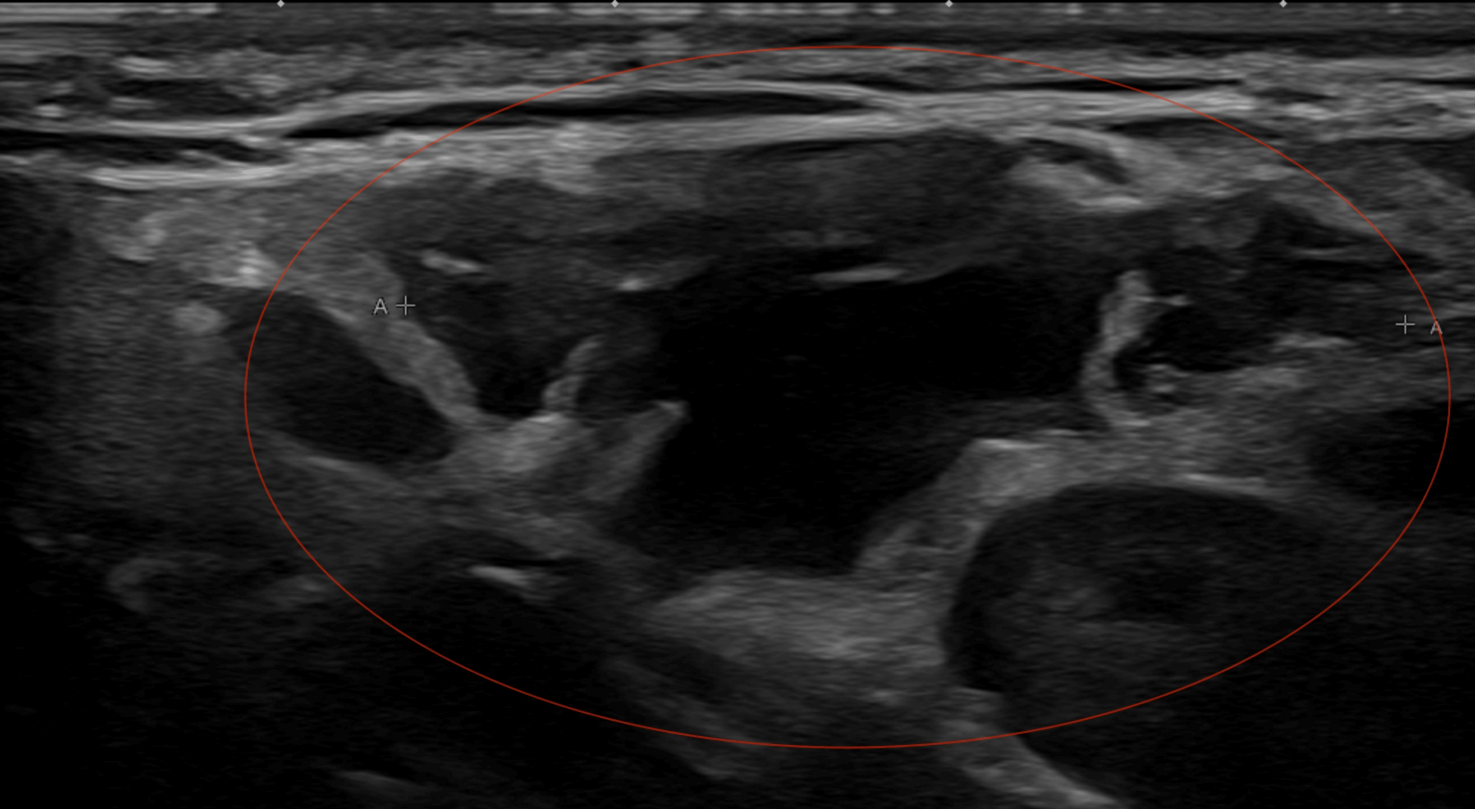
Ultrasound of the left upper neck demonstrating a multiloculated, avascular cystic lesion (circled in red) located between the parotid tail and the sternocleidomastoid muscle.
